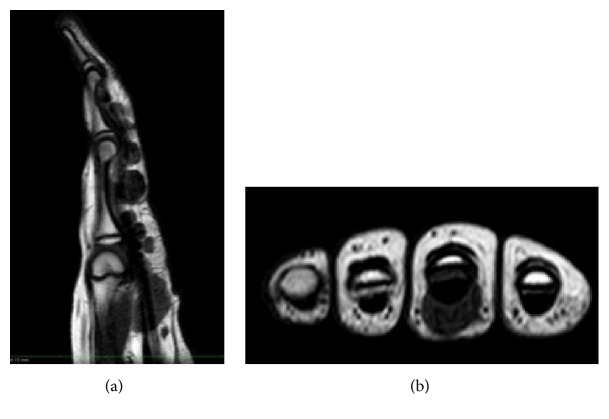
(a) MRI T1 sagittal image demonstrating hypodense masses on the volar aspect of the middle finger. (b) MRI T1 axial image demonstrating hypodense masses on the volar aspect of the middle finger.
